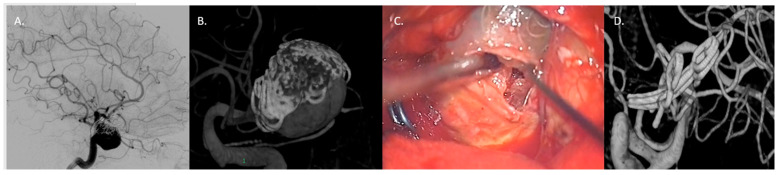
Case 4: complex clipping in previously coiled, giant, partially thrombosed 25 mm right MCA bifurcation aneurysm in a 76-year-old woman. (A,B) Preoperative presentation with DSA and 3D model of the previously coiled sac with a recurrence at 12 months. (C) Surgical clipping with progressive removal of thrombus and coils assisted by ultrasonic aspirator. (D) 3D reconstruction DSA confirming the complete exclusion of the sac.
